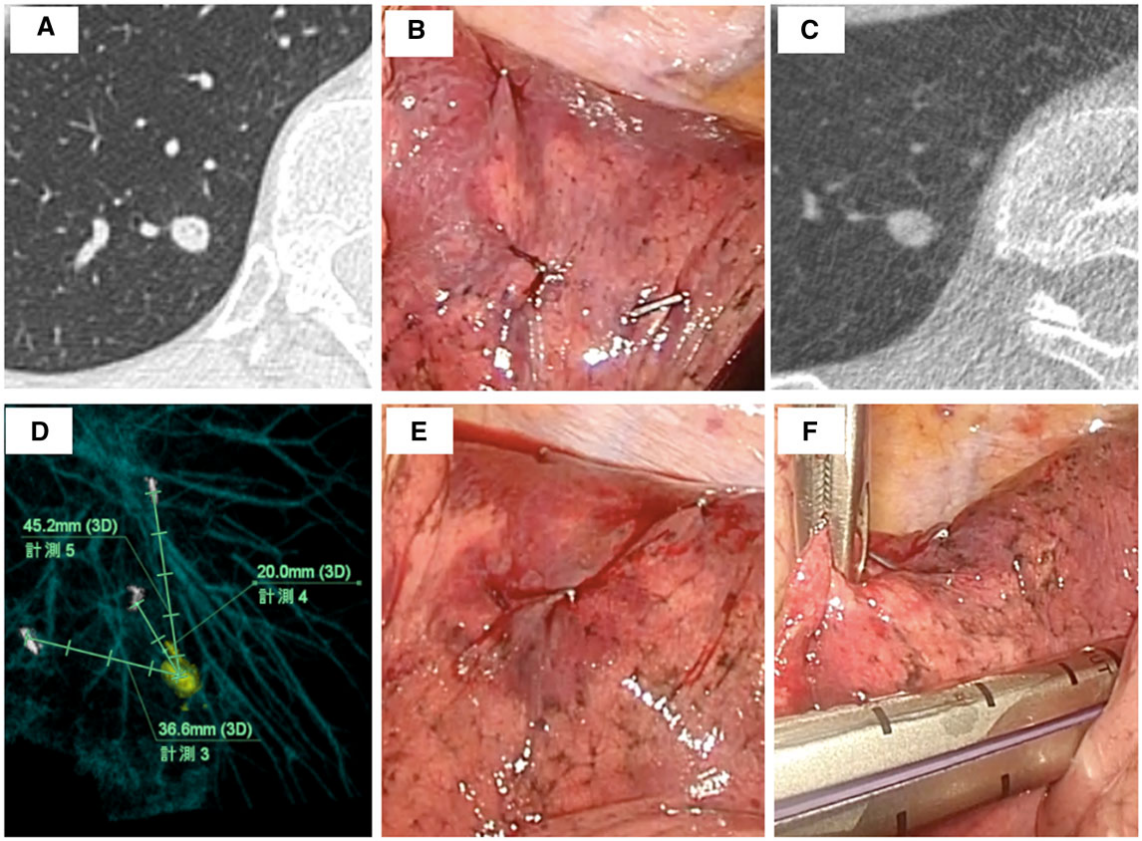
Procedure for localization of pulmonary tumour by cone-beam computed tomography. (A) Computed tomography image of nodule location. (B) Three surgical clips for first markers are attached to the visceral pleura around the suspected location of the referred tumour. (C) Cone-beam computed tomography image of a deep solid-type tumour. (D) Analysis of the location of the referred tumour. (E) One surgical clip for a second marker is attached to the visceral pleura above the referred tumour. (F) Thoracoscopic image showing wedge resection.
